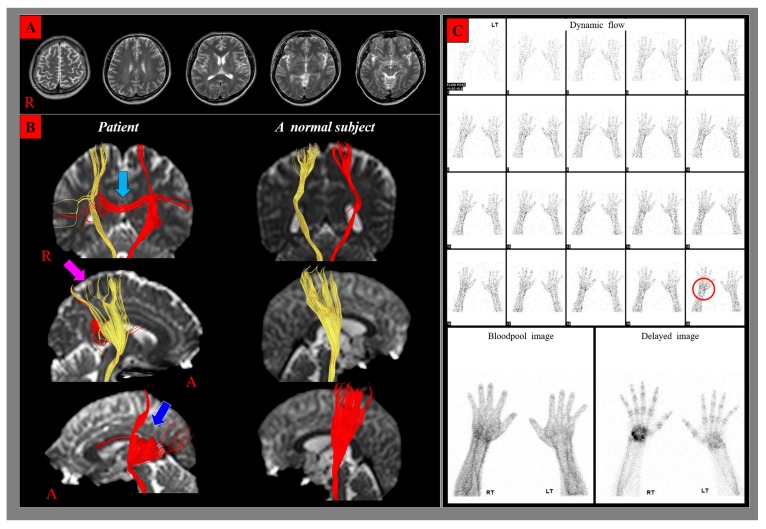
No specific lesion was observed on brain magnetic resonance images (T1-weighted, T2-weighted, and fluid attenuated inversion recovery images) taken at ten weeks after onset (A). On two-month diffusion tensor tractography (DTT), partial tearing of the CST was observed at the subcortical white matter in both hemispheres. The partial tearing of the CST was much more severe in the left CST (blue arrow) compared with the right CST (pink arrow) and transcallosal fibers (sky blue arrow) from the left corticospinal tract suggesting the CST injury was also observed (B). Plain X-ray, electromyography, and nerve conduction studies for the right upper extremity detected no abnormality. Three-phase bone scan showed that tracer distribution was higher in the right wrist at dynamic flow between the first and last image (red circle), and blood pool image. The delayed image revealed hot uptake in the right wrist and increased tracer uptake in the small joints of the right hand (C) [1,2,3]. (R: right, A: anterior, RT: right, LT: left).
